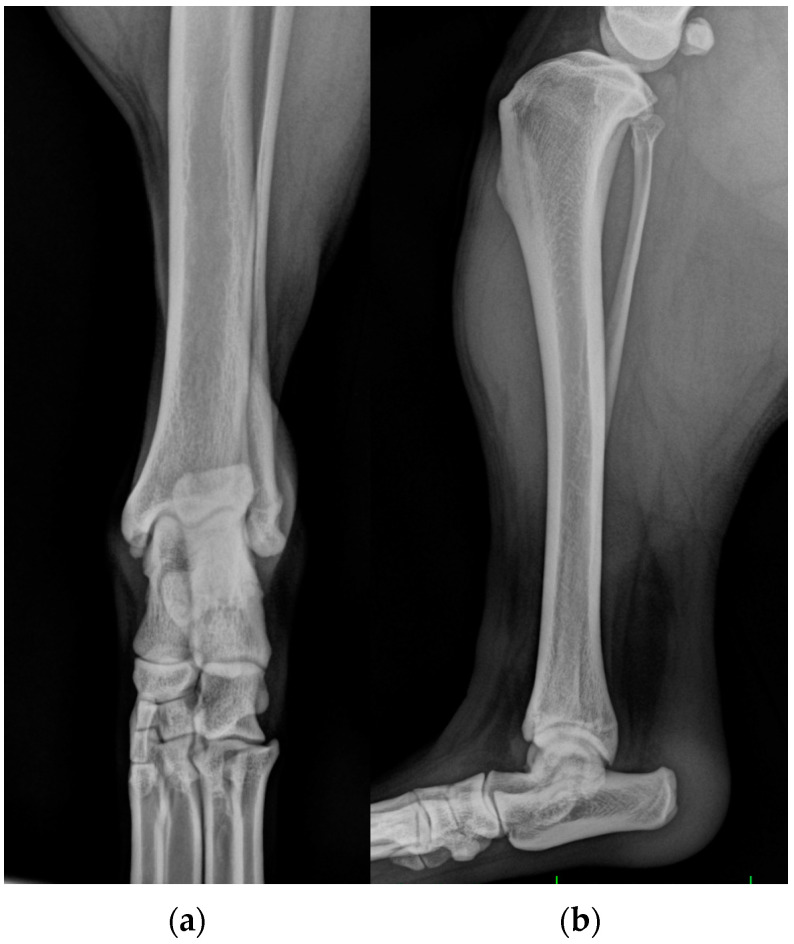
(a) Dorsoplantar and (b) mediolateral radiographic projections of the left tibial-tarsal joint showed soft swelling over the calcaneus without osteoarticular changes.
